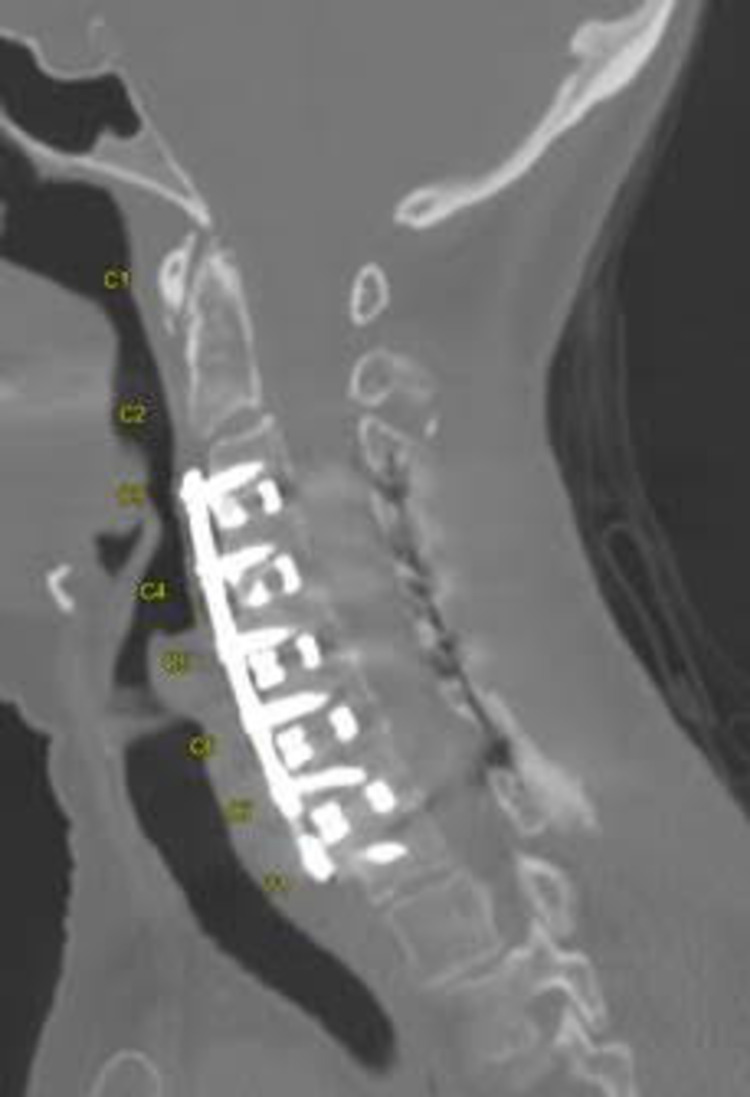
Midline sagittal CT scan of the cervical spine nine weeks after surgery Good bone healing without any screw lucency
